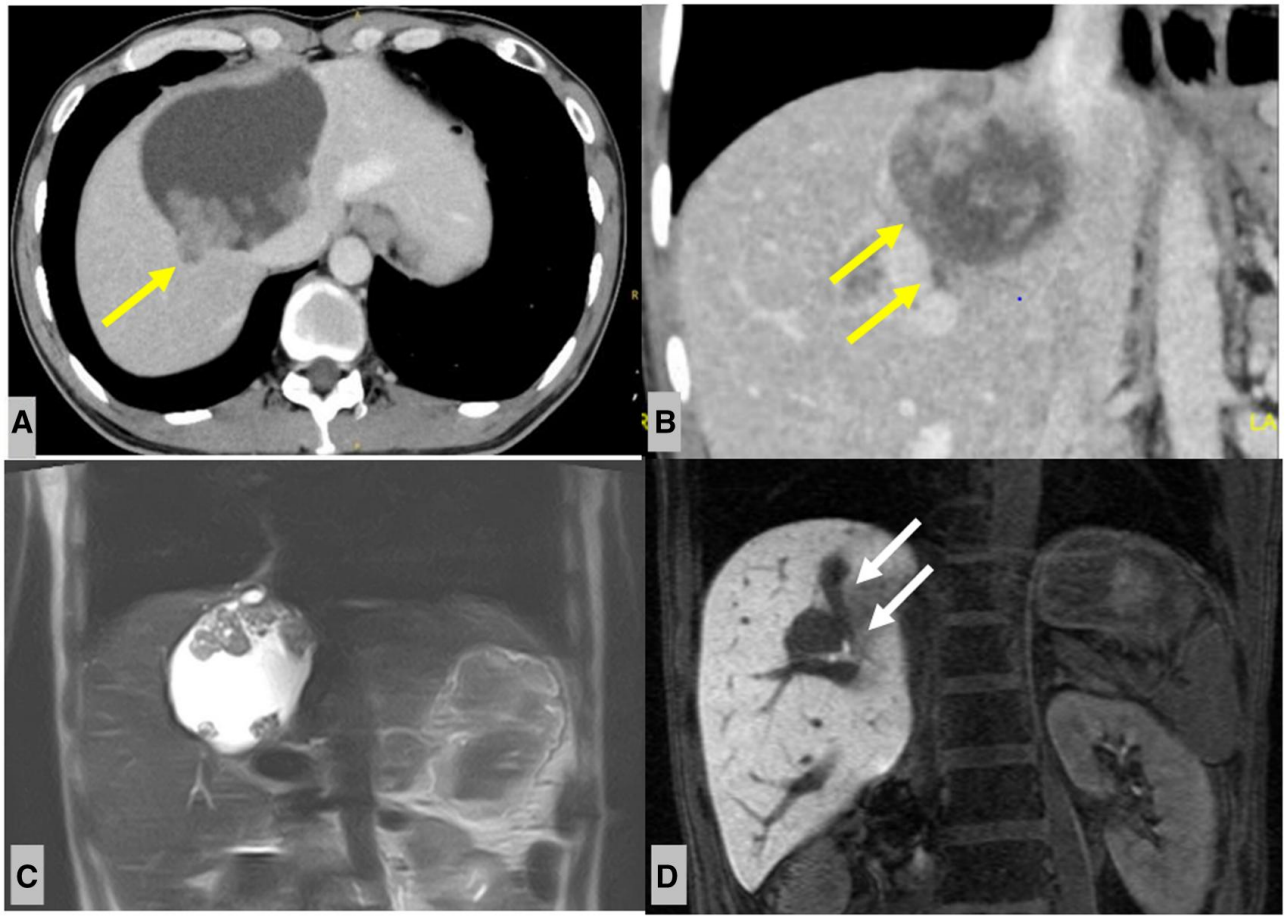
(A, B) Contrast-enhanced CT of the upper abdomen (one year after non-enhanced CT). Axial and coronal sections show several solid components in the hepatic cyst, and extension into the B7 bile duct (yellow arrow). (C) Coronal T2-weighted magnetic resonance image shows several solid components within the hepatic cyst. (D) Coronal hepato-biliary phase shows infiltration into intrahepatic bile duct B7, as suspected by CT imaging (white arrow). Abbreviation: CT = computed tomography.
